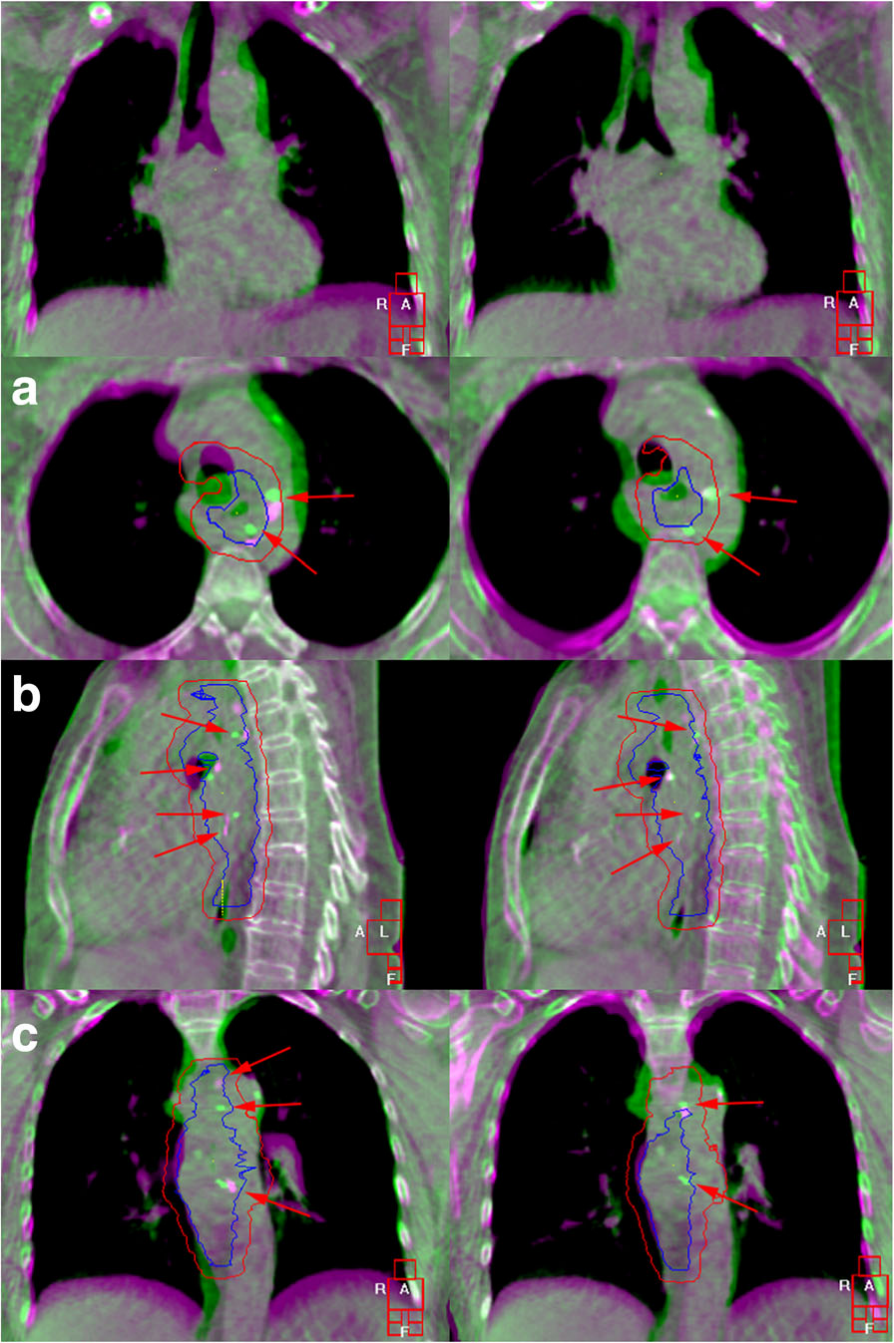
Overlay of the pCT (purple) and CBCT (green) in XVI for patient 9. Arrows indicate fiducial markers, registered on the bony anatomy (left column) and the carina (right column). In axial (a), sagittal (b), and coronal view (c). First row = general carina image in coronal view. A = anterior, R = right, L = left, and F = inferior. Red contour = PTV, blue contour = CTV
